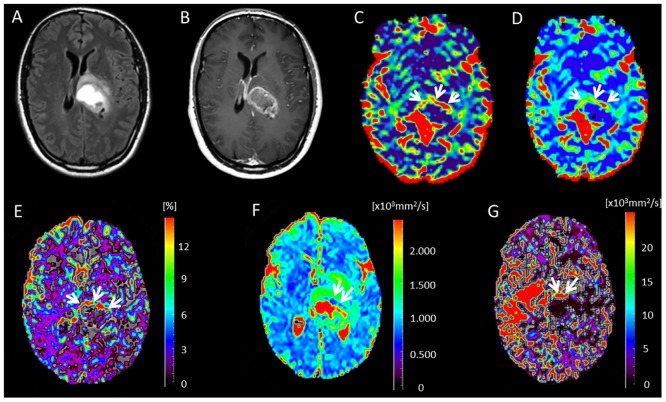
Glioblastoma in a 62-year-old woman. (A) Axial FLAIR image. (B) Contrast-enhanced T1-weighted image shows a rim-enhancing tumor. (C) Cerebral blood flow map obtained with DSC-MRI shows hyperperfusion signals predominantly in the left margin of the tumor (*arrows*). (D) DSC cerebral blood volume map. The hyperperfusion signal intensities correlate with those seen on cerebral blood flow map. (E) *f* map clearly highlights the area with high perfusion in the margins of the tumor (*arrows*), which is more evident than in C and D. (F) *D* map shows small restricted diffusion area (average *D* value = 0.895 x 10^−3^ mm^2^/s) predominantly in the anterior tumor margin (*arrows*). (G) *D map shows increased fast-diffusion values in the tumor tissue (arrows).
